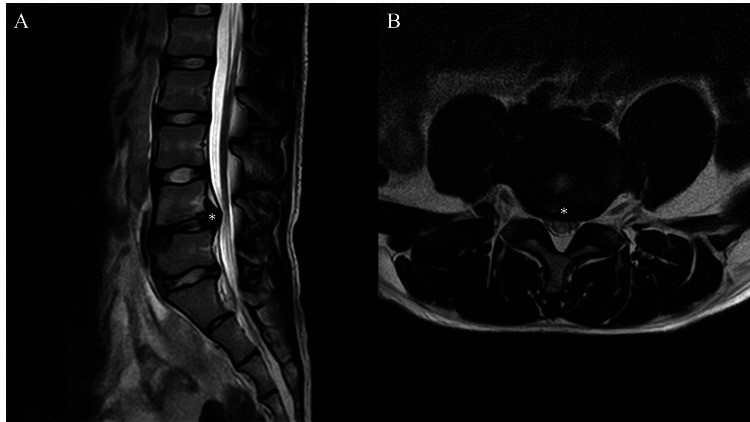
Magnetic resonance imaging Magnetic resonance imaging confirmed the herniation of the intervertebral disc between the fourth and fifth lumbar vertebrae (asterisk). A: sagittal plane, B: axial plane
